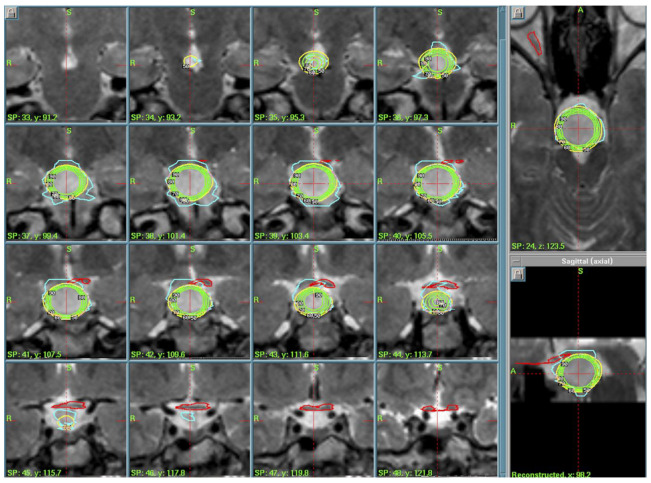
Case 1 treatment and dose-planning in a coronal T2-weighted MRI. In yellow is the 50% isodose line prescribed to the lesion, and in green are the 60% to 90% isodoses. In green and light blue are the contours of the anterior optic pathways and pituitary gland and pituitary stalk.
